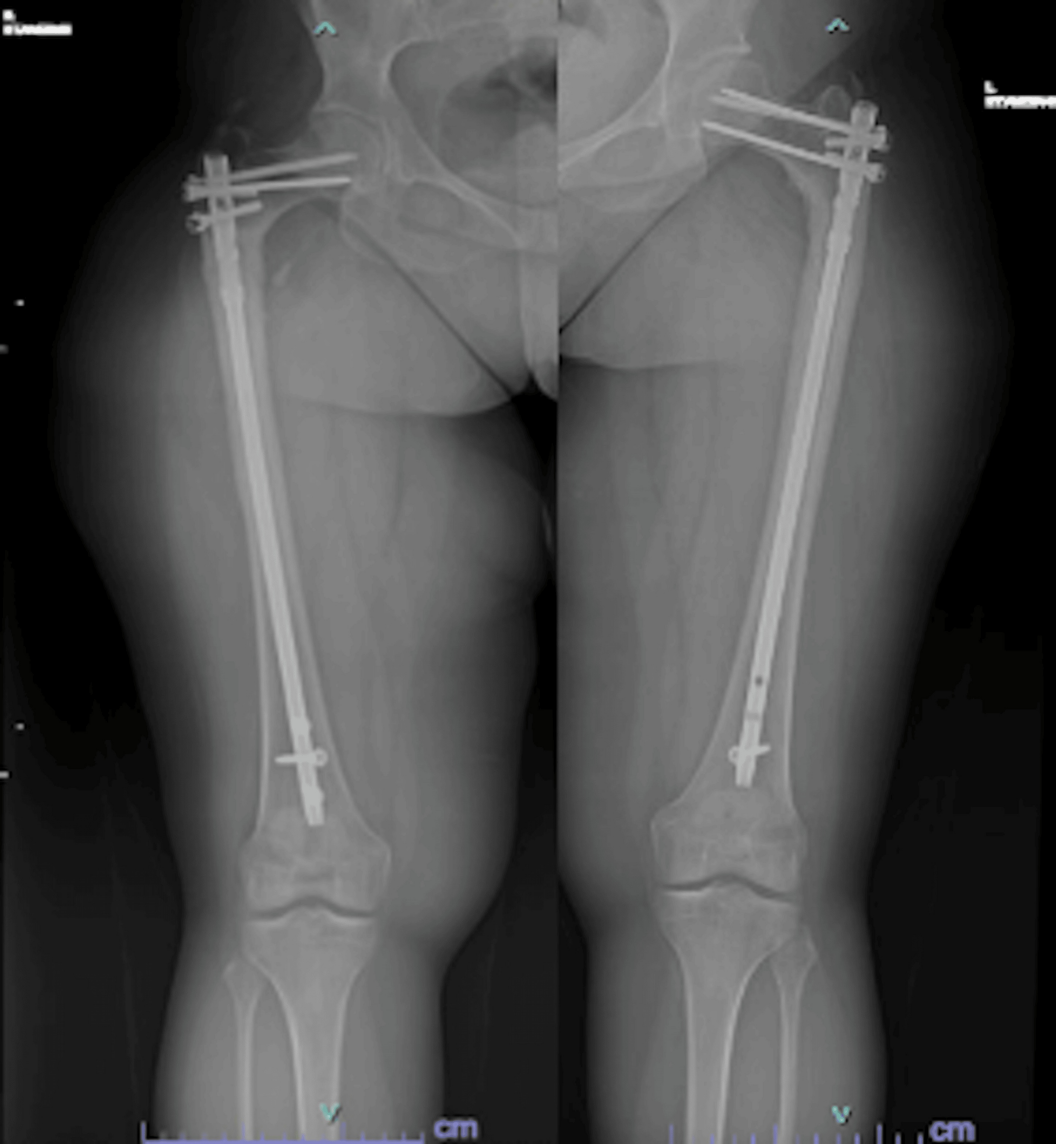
Most recent imaging showing radiographically healed fracture and intact hardware
